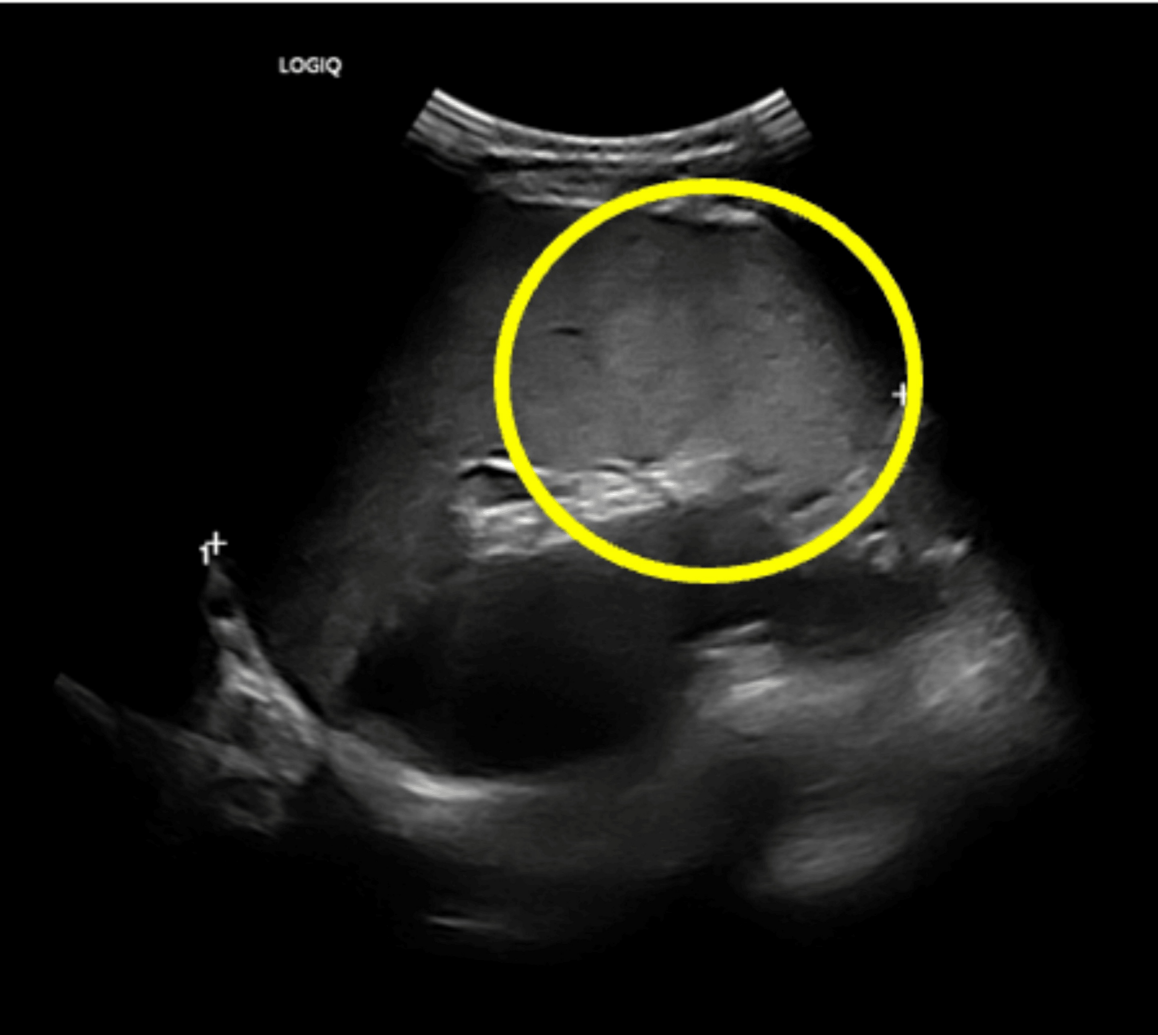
Abdominal ultra sonography The marked region shows splenomegaly.
